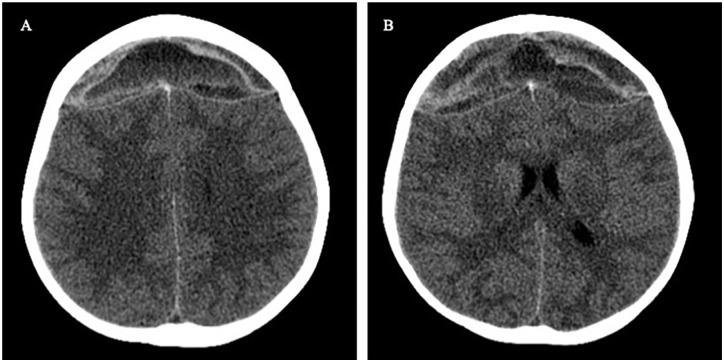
(A and B) Initial axial head computed tomography without contrast demonstrates
